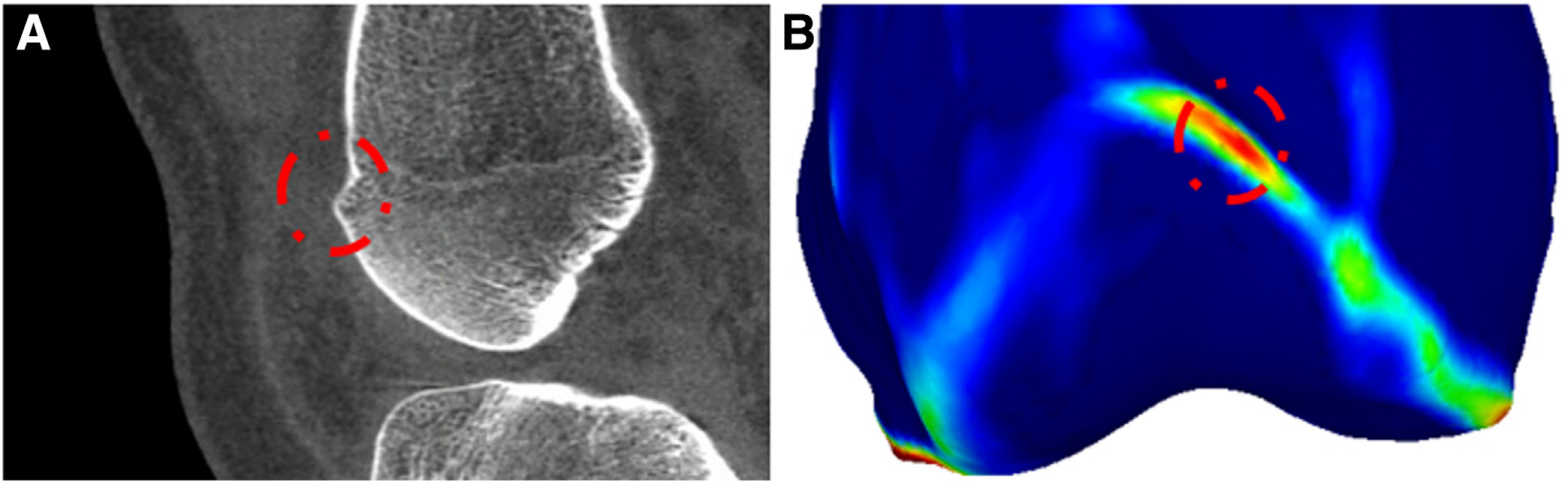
Highlighting of proximal trochlear ridges. (A) A computed tomography scan of a patient with a lateralized medial ridge. (B) The corresponding 3-dimensional model with curvature visualization. The trochlear ridge is highlighted in green to red, with cold colors indicating negative to low maximal curvature and warm colors indicating high curvature.
